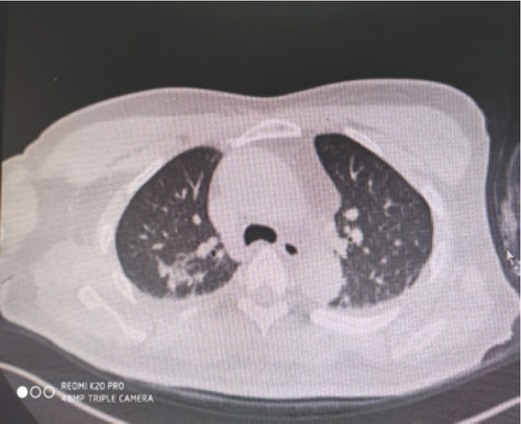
Lung CT performed on 24 October 2019, seven days after the accident, showing a few regions of inflammation in both lungs, fibrous foci, a small amount of effusion in the bilateral pleural cavity, and swelling of adjacent pulmonary tissue
